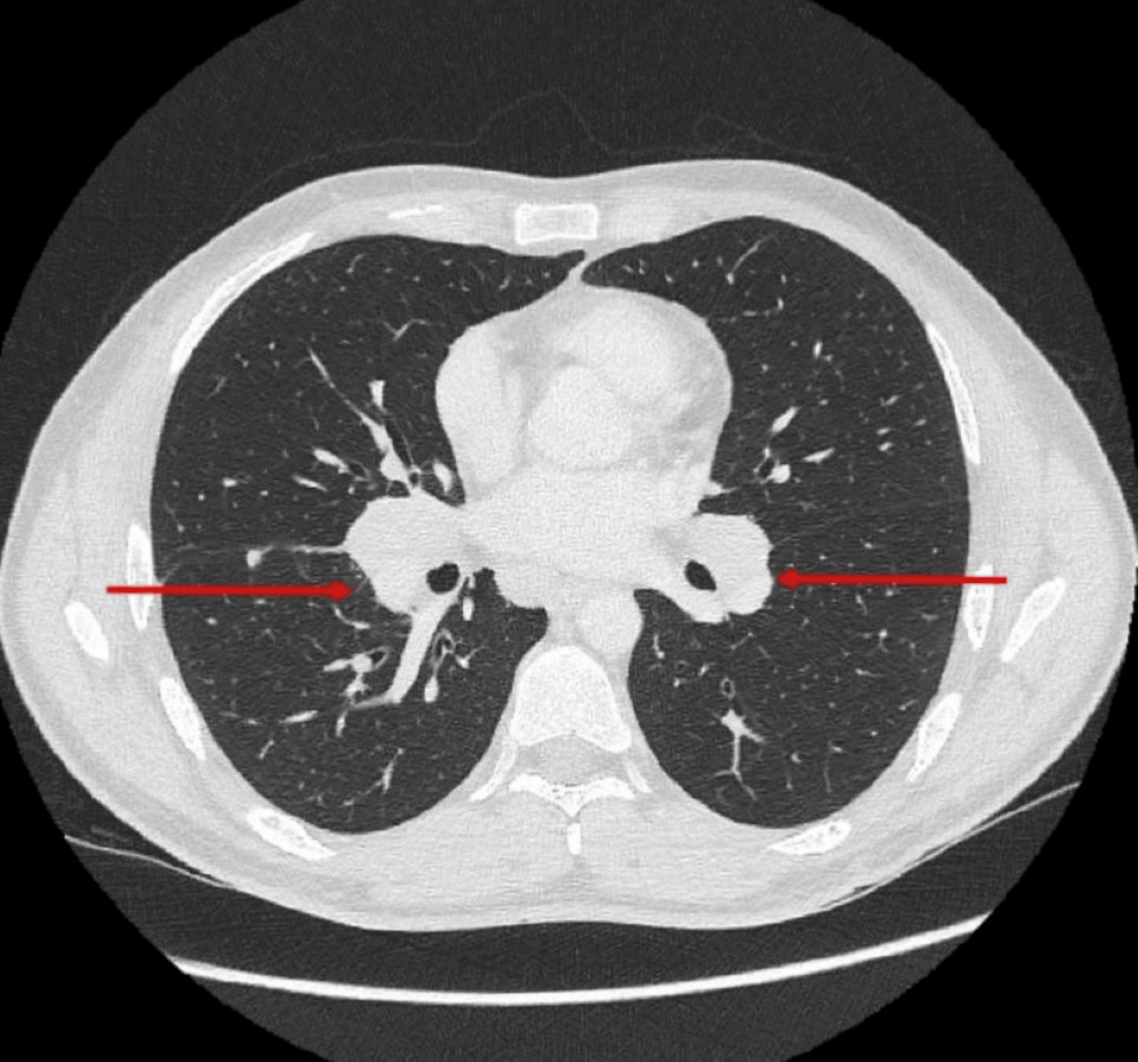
Chest CT Chest CT showing bilateral hilar lymphadenopathy (red arrows).
